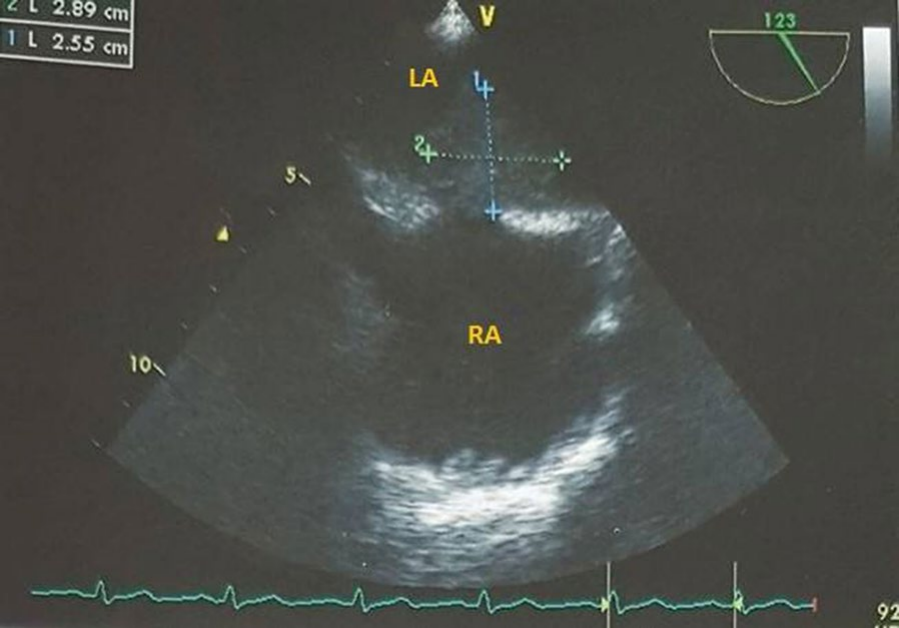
Transoesophageal echocardiography showing mid esophageal 20° view. Left atrium tumor. *RA* right atrium, *LA* left atrium
